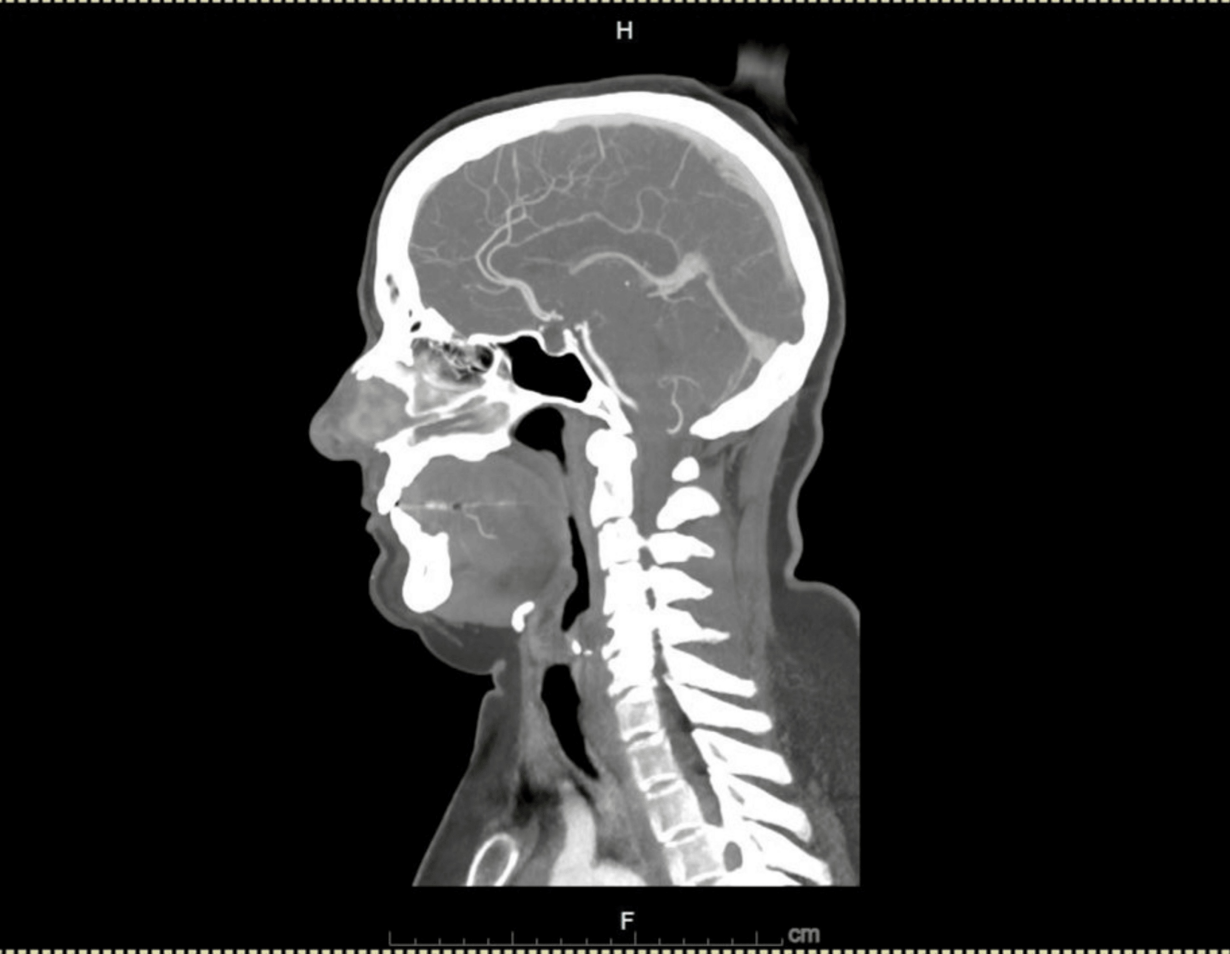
Computed tomography angiography (CTA) sagittal view of head and neck showing normal patent arteries
